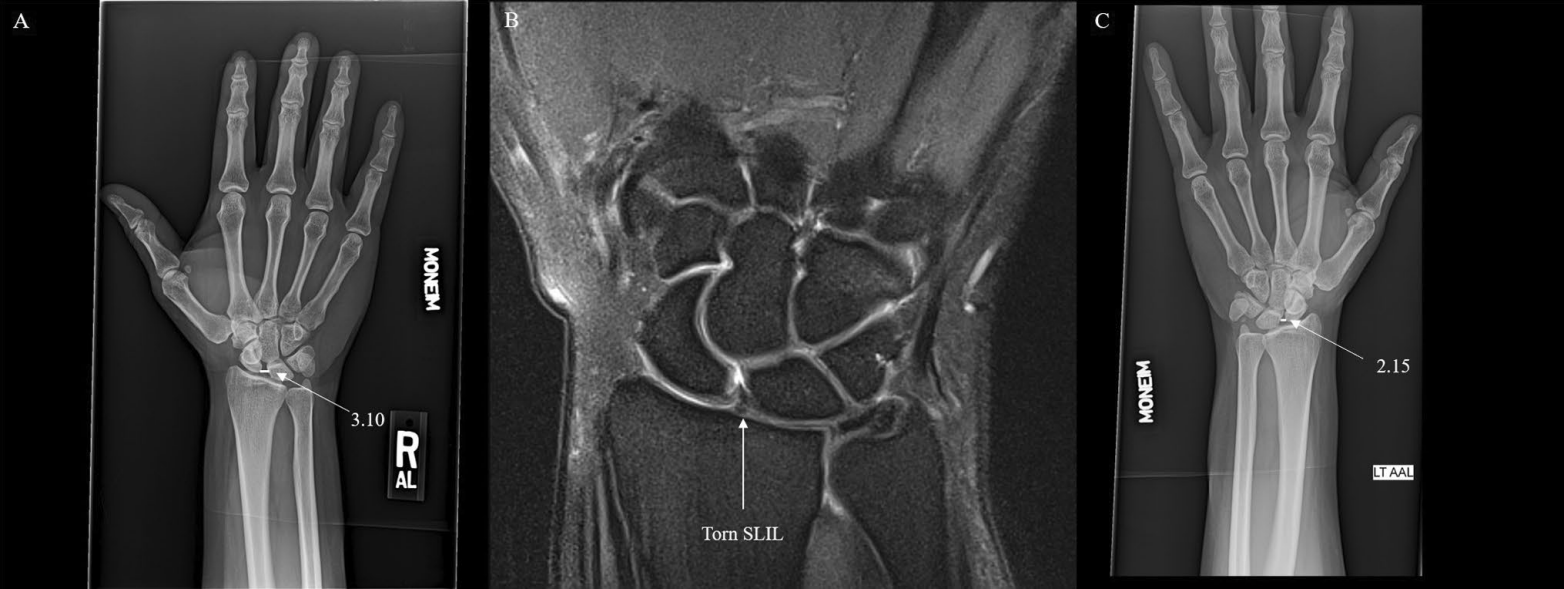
A-Radiograph of tangential Moneim view of injured wrist with average SL gap of 3.10 mm. B: Coronal Proton Density Turbo Spin Echo (PD TSE) MRI showing torn SLIL. C-Radiograph of tangential Moneim view of normal wrist with average SL gap of 2.15 mm
